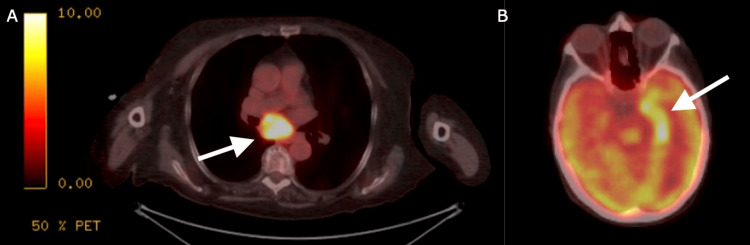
PET CT showing (A) a soft tissue mass in the lung suspicious for malignancy and (B) left hippocampal hypermetabolic activity suspicious for possible paraneoplastic encephalitis.
